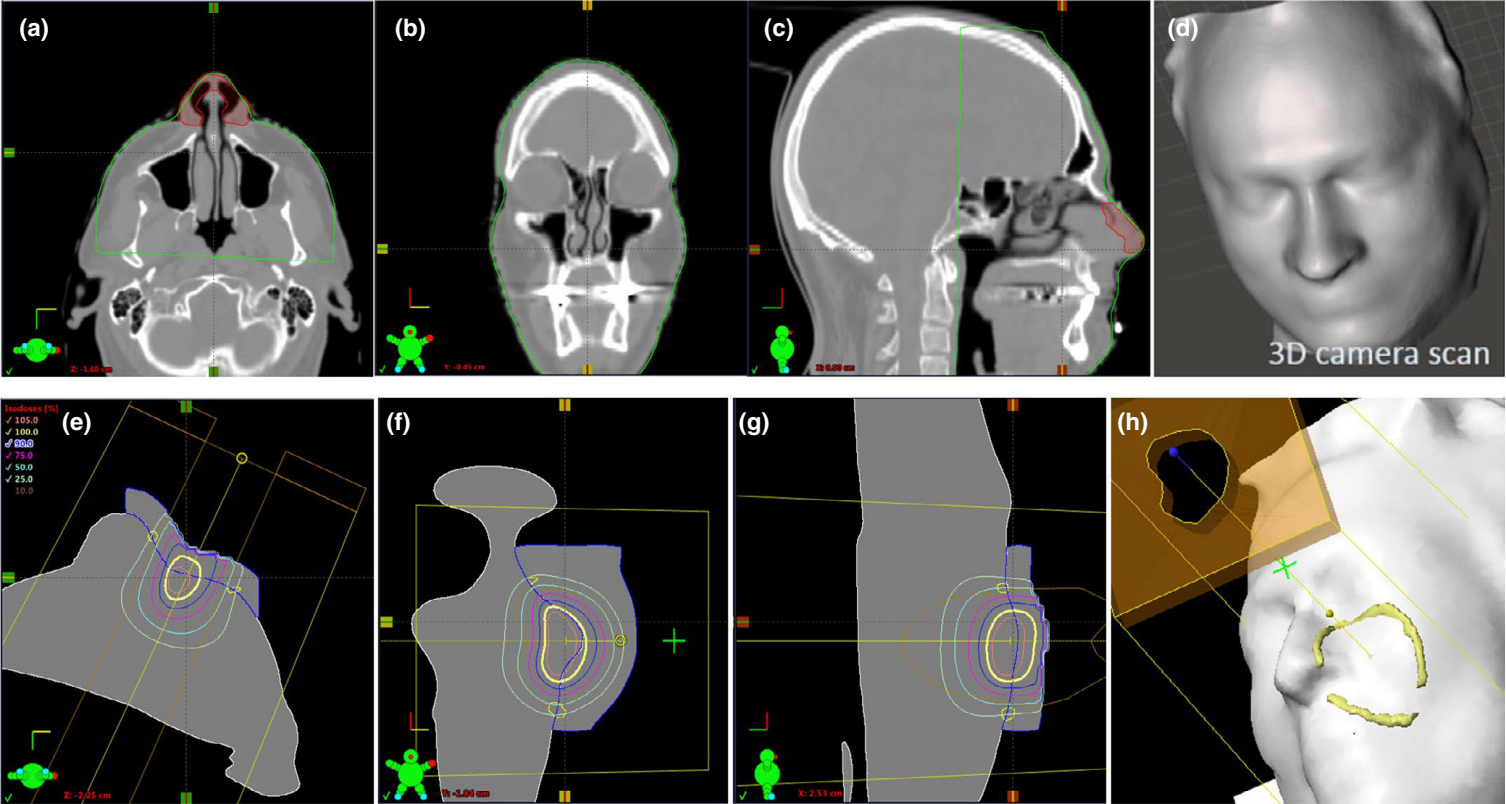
(a–d) 3D camera captured contours (green line) with its full CT scan demonstrate the 3D camera accuracy on a human patent. (e–g) No CT scan was performed, only a 3D camera scan. The bolus was created using the 3D camera captured contours. (h) The yellow outline is derived from the colormap in the 3D camera scan which was used to segment the field borders that were defined with ink marks on the patient’s face by the physician.
